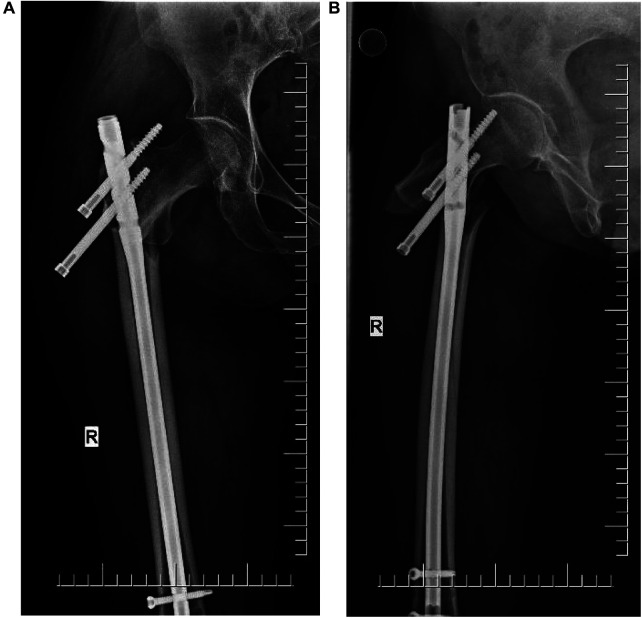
(A,B) X-ray (2 months after surgery). The proximal end of the nail was dislodged, and the broken end was displaced again.
